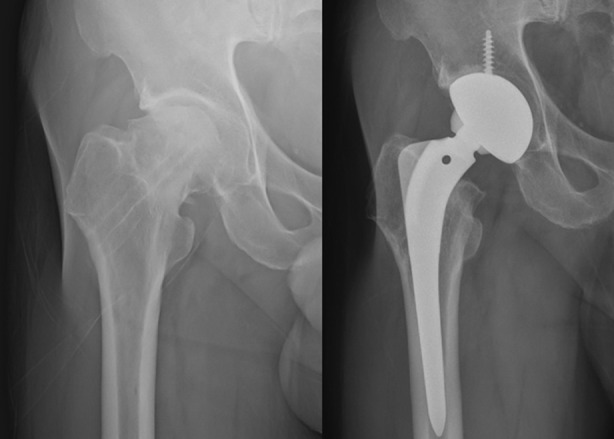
This patient is 63-year-old male. The radiograph taken 22 months after hardware removal show avascular necrosis of right-side femoral head. So we performed total hip replacement arthroplasty.
